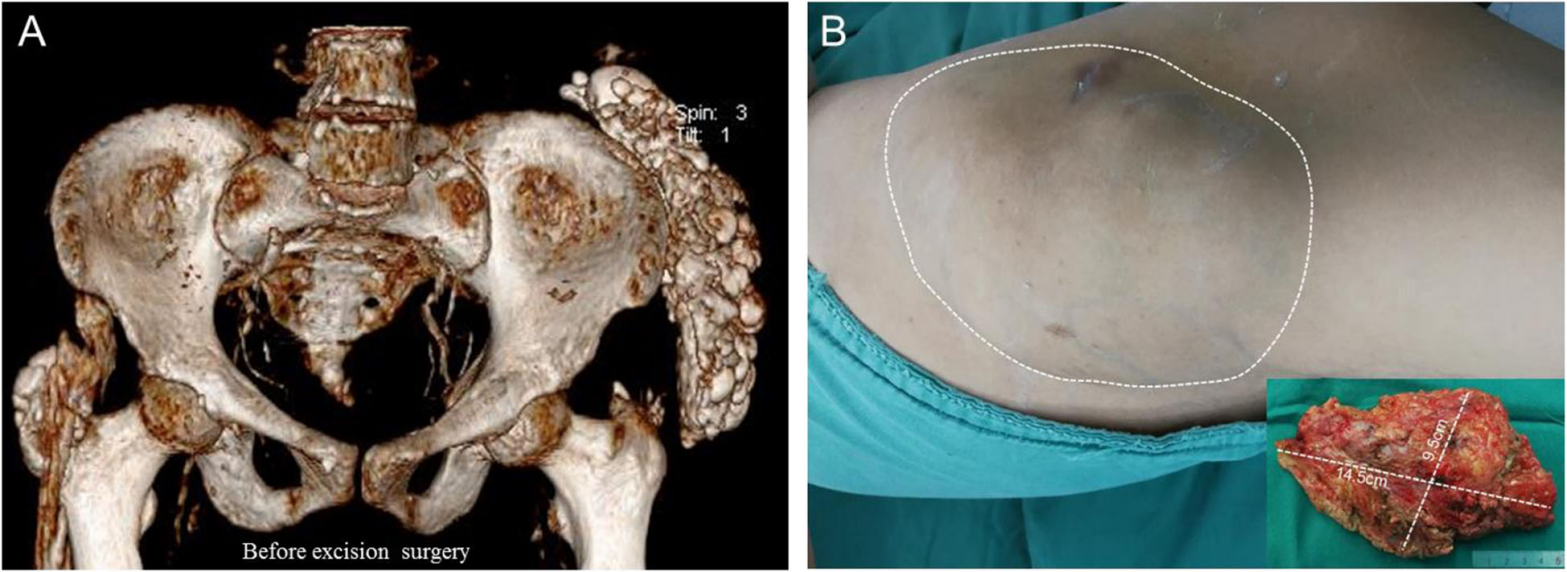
Surgical excision of mass in case 2. a CT scans with 3D reconstructions showed remarkable ectopic calcifications in the left buttock that limited left-hip mobility. b The size of the mass resected from the left buttock was 14.5 × 9.5 cm^2^
